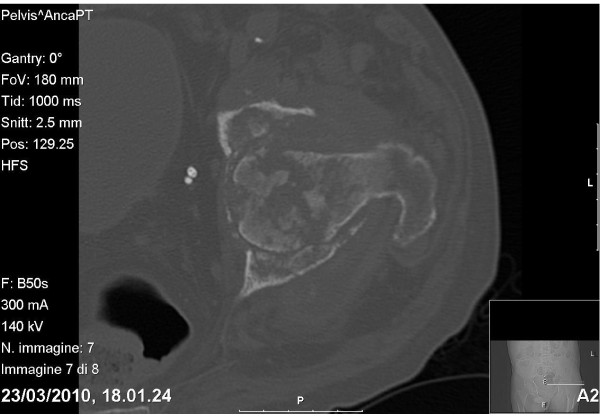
CT image of the coxofemural joint showing extensive bone resorption.
